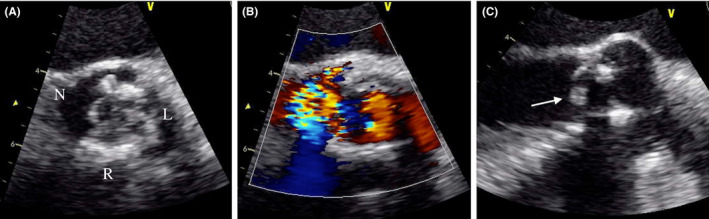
Preoperative transesophageal echocardiography. A, B, Severe aortic regurgitation at the torn noncoronary cusp (R: right coronary cusp, L: left coronary cusp, N: noncoronary cusp), and (C) a torn, prolapsed noncoronary cusp (white arrow)
